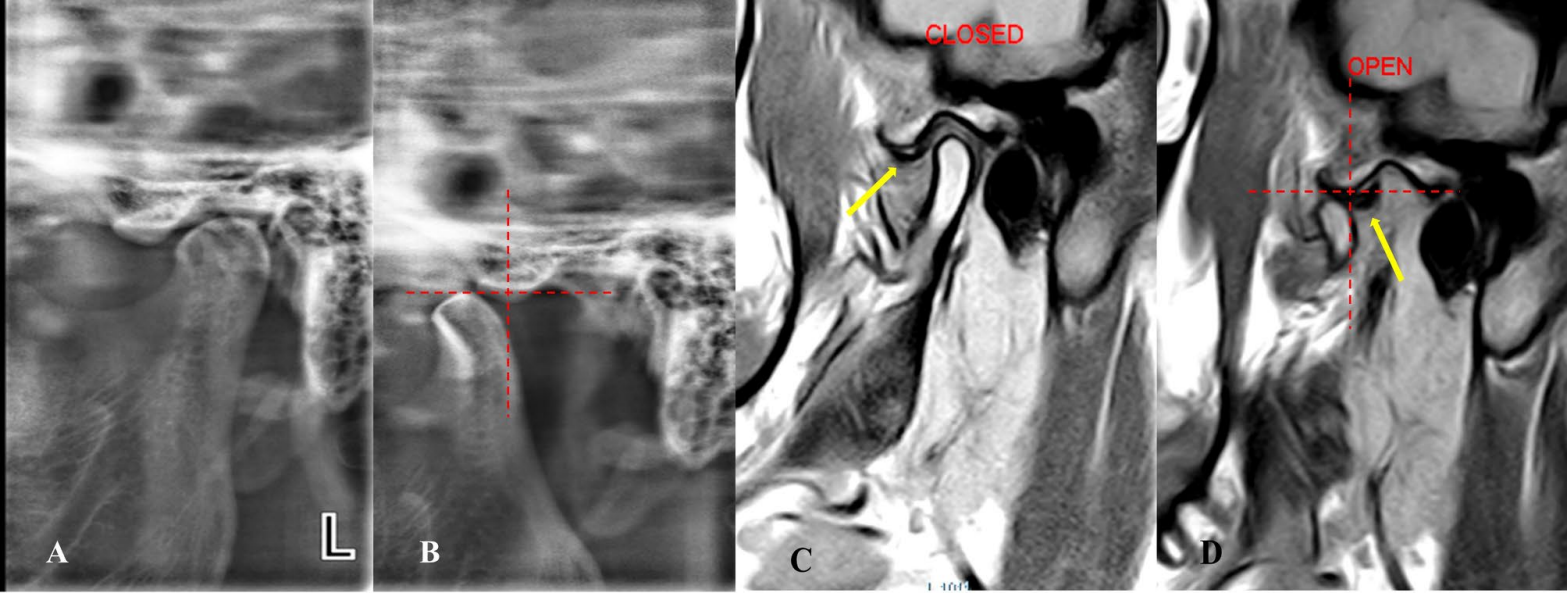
Lateral TMJ view represent Stage I-B in closed (A) mouth position & in open (B) mouth position showed the condyle is located anterior to the eminence but at the same level of the highest point of the eminence. Oblique sagittal T1-weighted images representing stage I-B showed anterior disk displacement (yellow arrow) in closed (C) mouth position with recapture of the articular disk (yellow arrow) during open (D) mouth position. The position and the disk-condyle complex position at the same level of the highest point of the eminence
